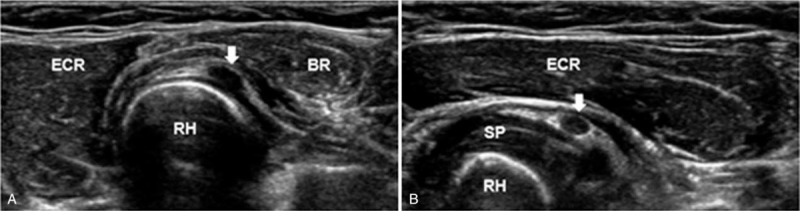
Axial A, and longitudinal B, ultrasonographic findings of the patient's right proximal forearm, showing a hypo-echoic, well demarcated ganglion cyst (arrows) located on the anterolateral side of the radial head. BR = brachioradialis, ECR = extensor carpi radialis, RH = radial head, SP = supinator.
